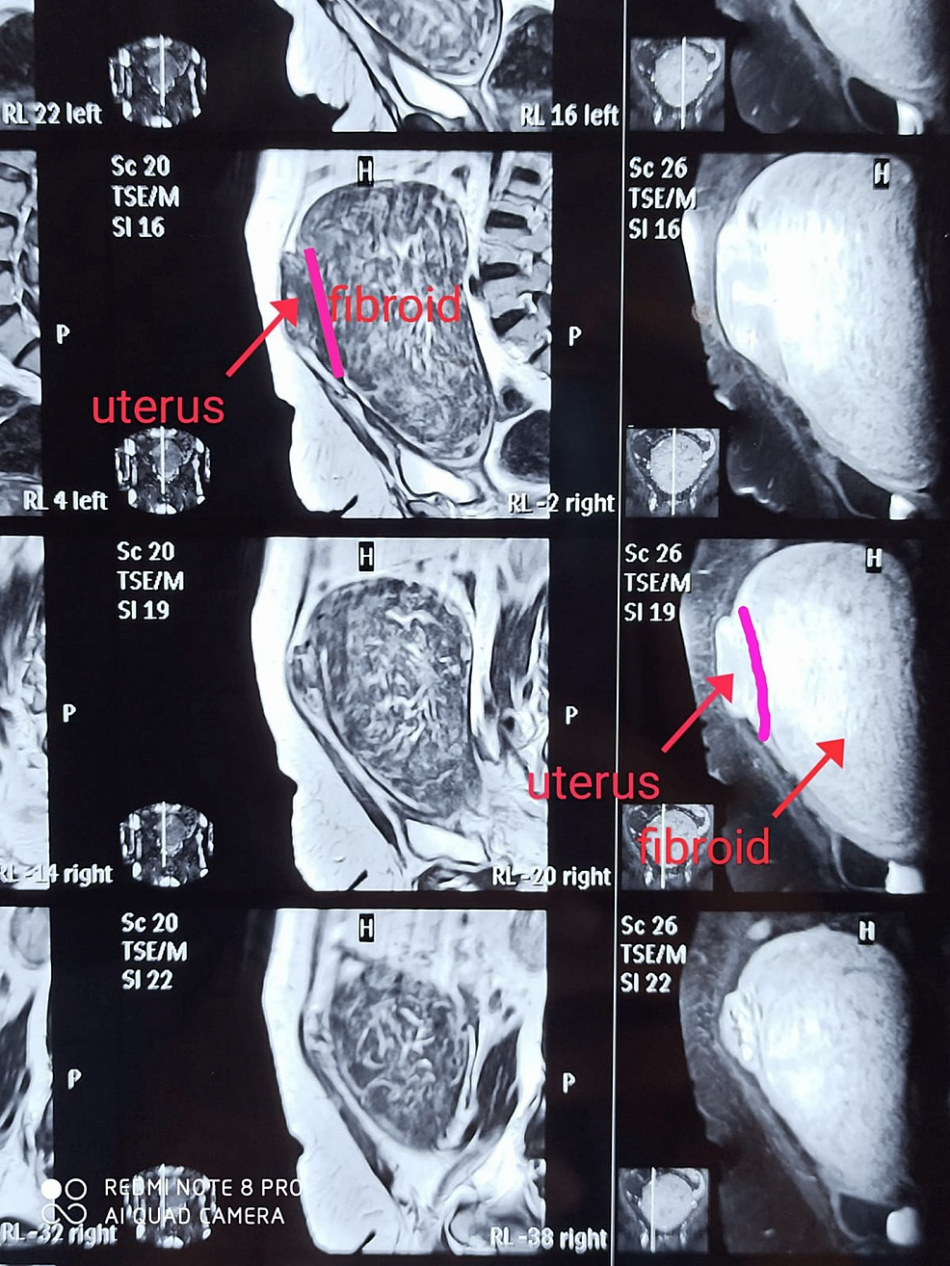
MRI showing large cervical fibroid
